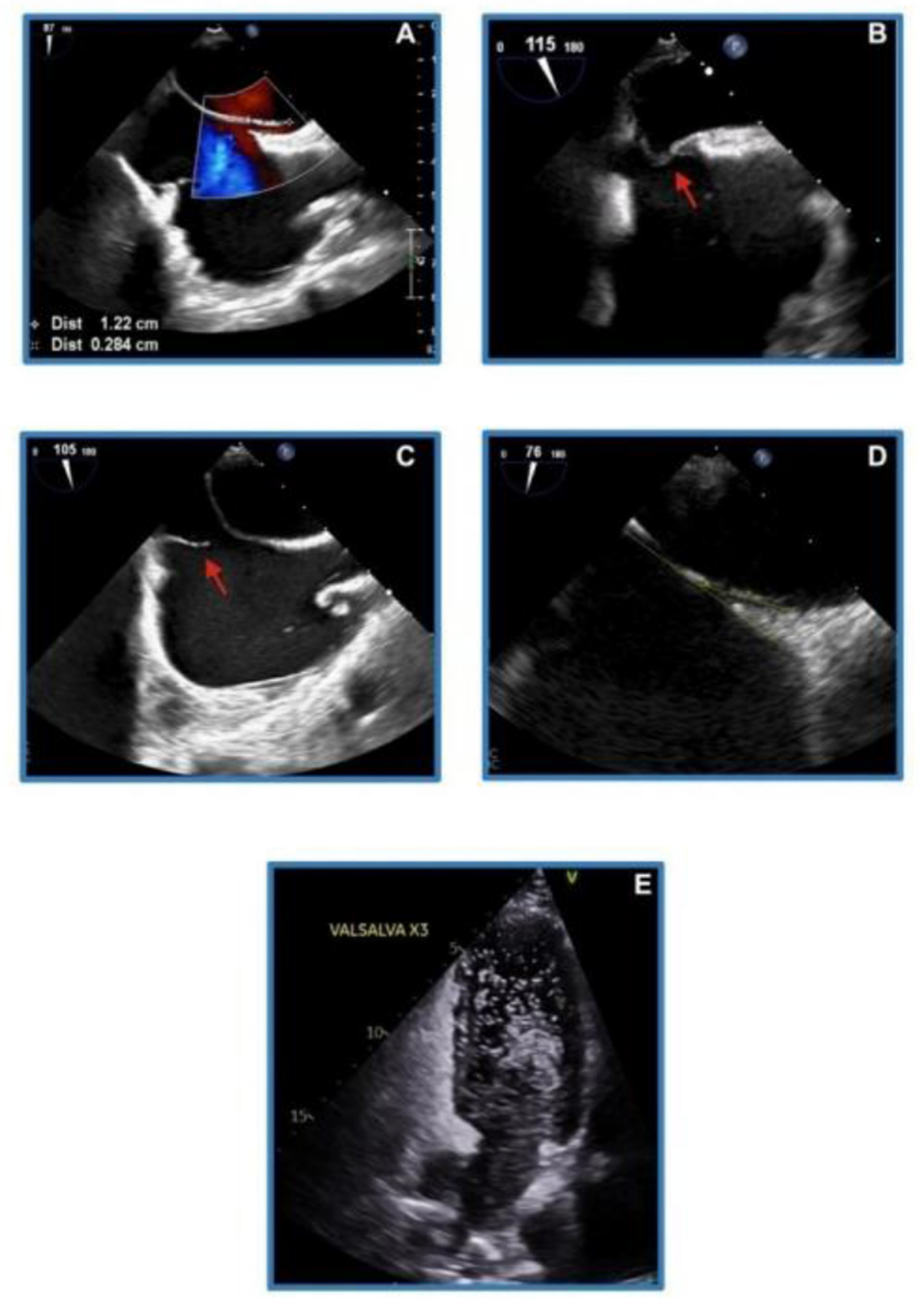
High-risk PFO anatomical features. (A) Measurement of the PFO showing a height (maximum separation between septum primum and septum secundum) >2 mm, and a tunnel length (maximum overlap) >10 mm. (B) Aneurysmal interatrial septum, defined as septal excursion ≥10 mm during the cardiac cycle. (C) Prominent eustachian valve, defined as a linear structure protruding ≥10 mm into the right atrium. (D) Low-angle PFO configuration, characterized by a sharp angle (< 10°) between the inferior vena cava (IVC) inflow and the PFO tunnel, assessed in an imaging plane containing both the IVC and interatrial septum. (E) Large right-to-left shunt on bubble transthoracic echocardiogram, defined as >20 microbubbles appearing in the left atrium within three cardiac cycles of right atrial opacification. *Note*
